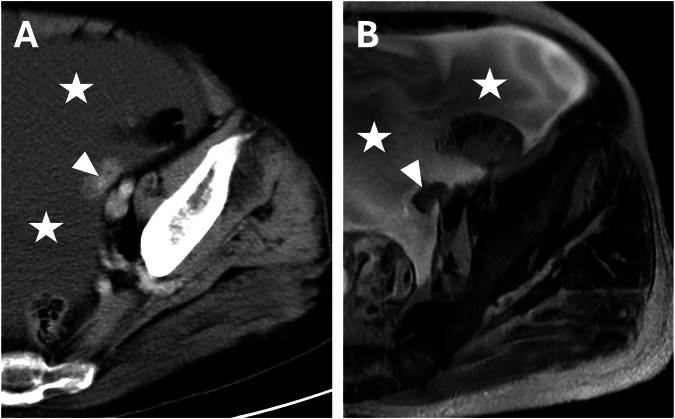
Colon adenocarcinoma with peritoneal carcinomatosis in an 81-year-old woman. CT portal-venous phase (A) and MRI T2w (B) demonstrated a nodular pattern in the left iliac fossa (arrowheads) and diffuse ascites (stars)Calcification: manifesting as areas of hypointensity on MRI scans, both on T1 and T2-weighted sequences and regions of hyperattenuating on CT scan (Fig. 5). Calcified peritoneal implants may suggest a primary ovarian serous cystadenocarcinoma, a primary papillary mucinous carcinoma, or as a result of chemotherapy [27].
